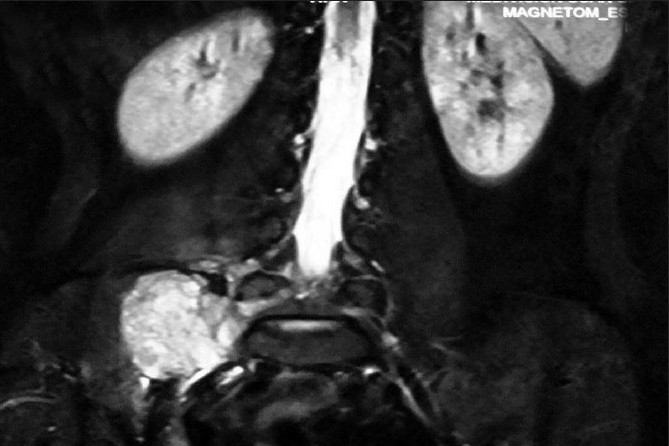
T1-weighted MRI showing a tumor mass at L5-S1 region
